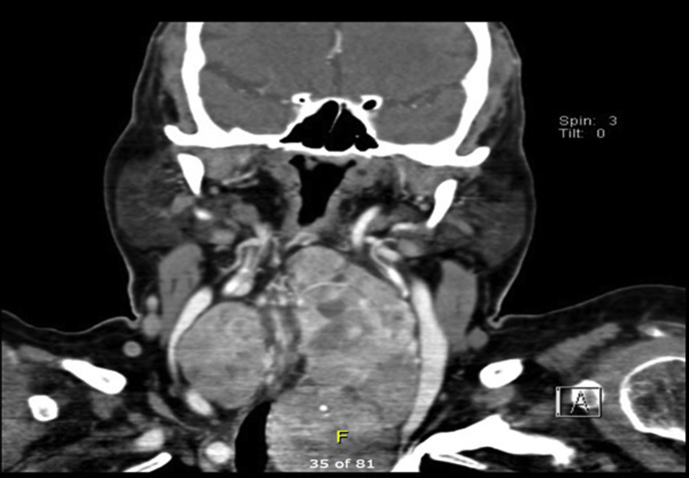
CT with contrast, Coronal view demonstrating heterogeneous left thyroid lobe enlargement with retrosternal extension.
